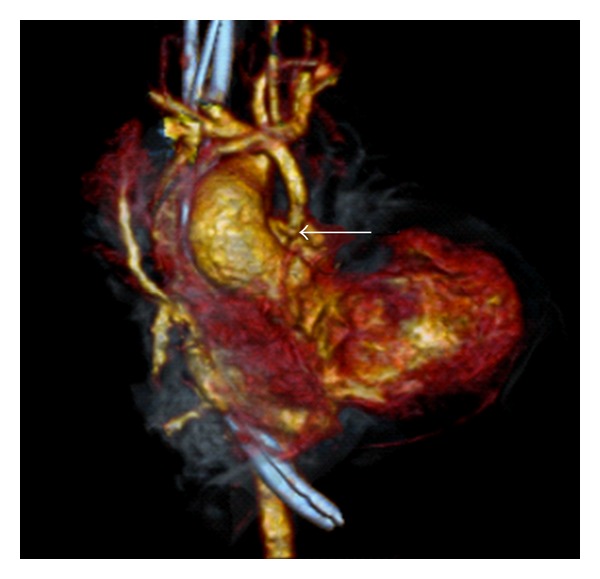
CT angiography with 3-dimensional reconstruction demonstrating a narrowing of the modified BT shunt at the point of anastamosis with the pulmonary artery.
